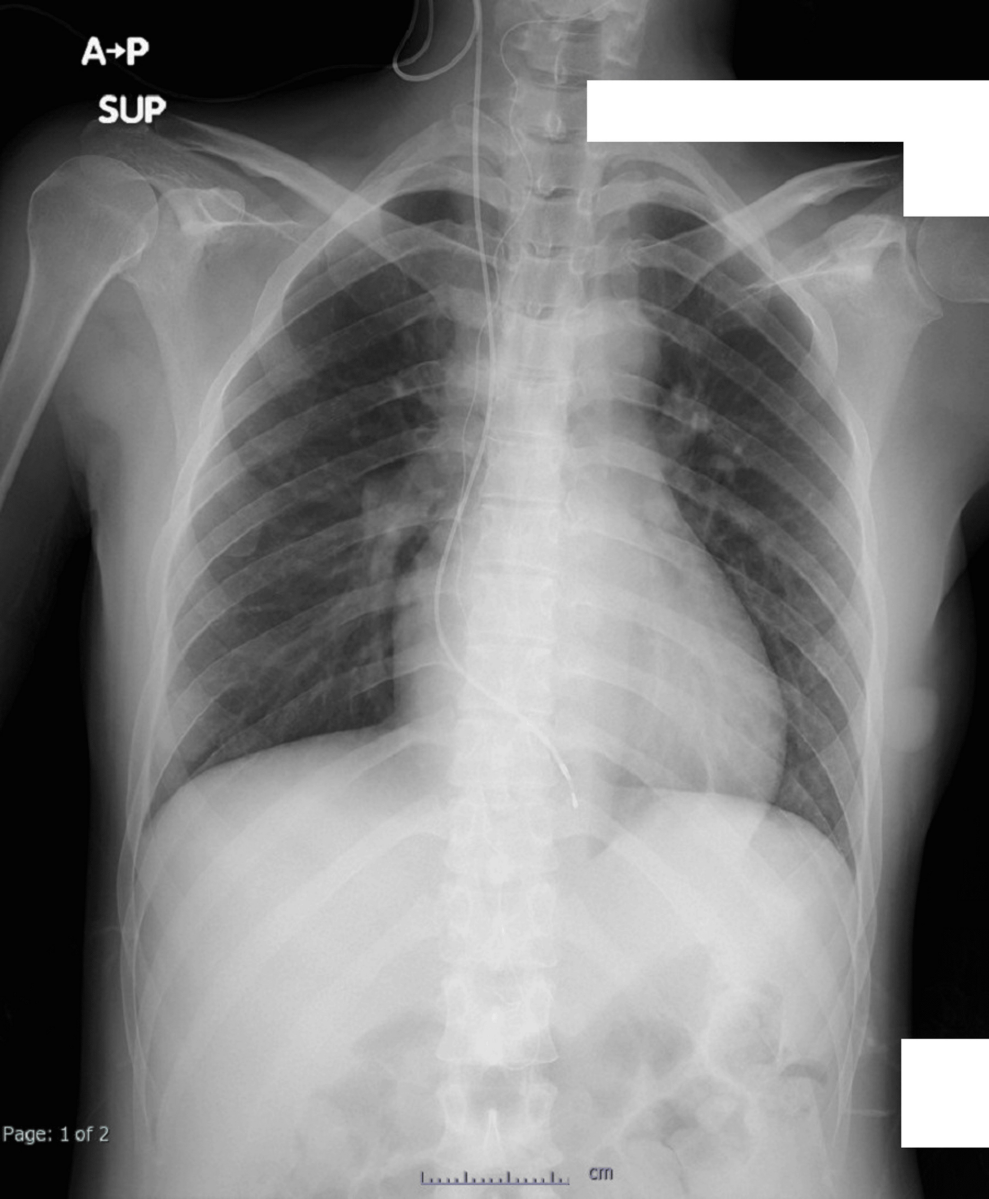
Chest X-ray taken after temporary pacemaker was inserted via right internal jugular vein to the right ventricle.
